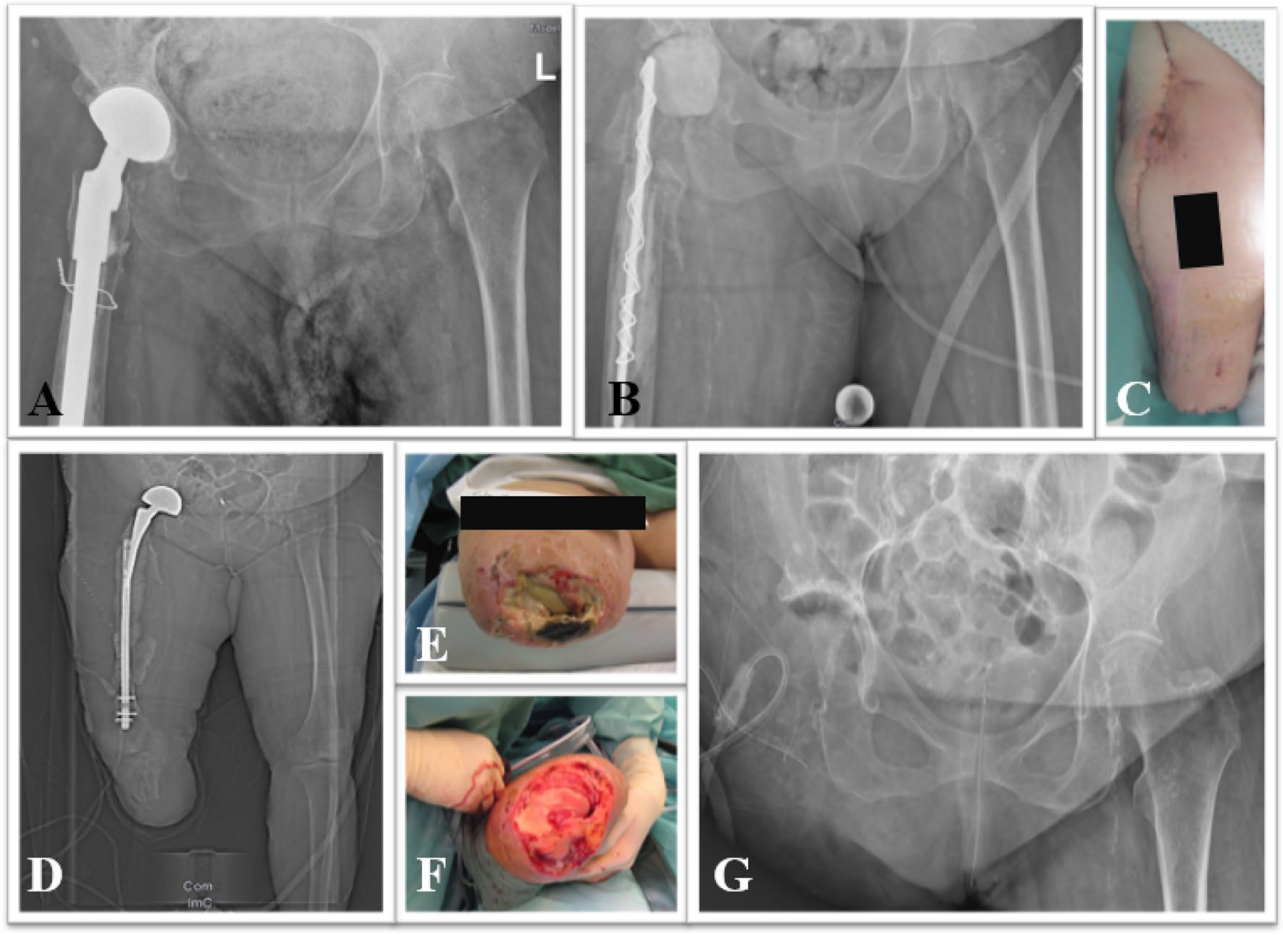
66 year-old female patient with FRI after open reduction and internal plate fixation distal fibula fracture, end-stage peripheral artery disease and consecutive concomitant PJI of the hip. A X-ray of the pelvis with revision endoprosthesis prior to explantation (a.p. view). Puncture revealed vancomycin-resistant *Enterococcus faecalis*. B Postoperative X-ray after implantation of a PMMA spacer. Below knee amputation was indicated due to severe ankle osteomyelitis and lateral soft tissue defect. C Image after below knee amputation. D CT scout after below knee amputation. E Infection of the amputation stump after three months. F Intraoperative image after debridement. Due to persistent wound infection and soft tissue defect hip discarticulation was performed. G Postoperative X-ray after hip disarticulation
